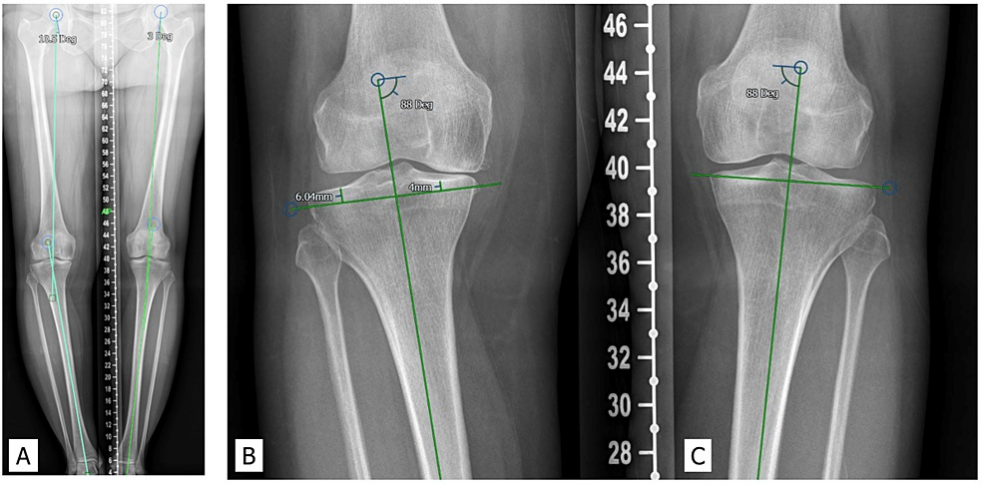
A: X-ray shows how to measure the hip-knee-ankle angle; B and C: Anatomical resection for a patient
